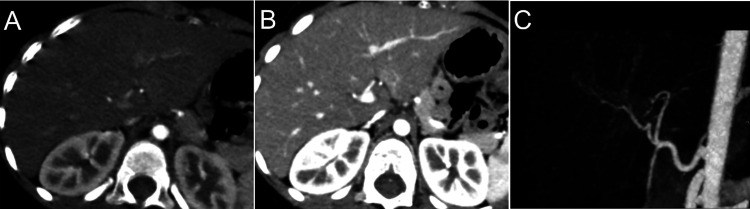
Second follow-up contrast-enhanced computed tomography performed 23 days following the injury (A) Axial view of CECT in the early arterial phase, (B) axial view of CECT in the late arterial phase, and (D) three-dimensional CT angiography, showing hepatic pseudoaneurysm disappearance.
